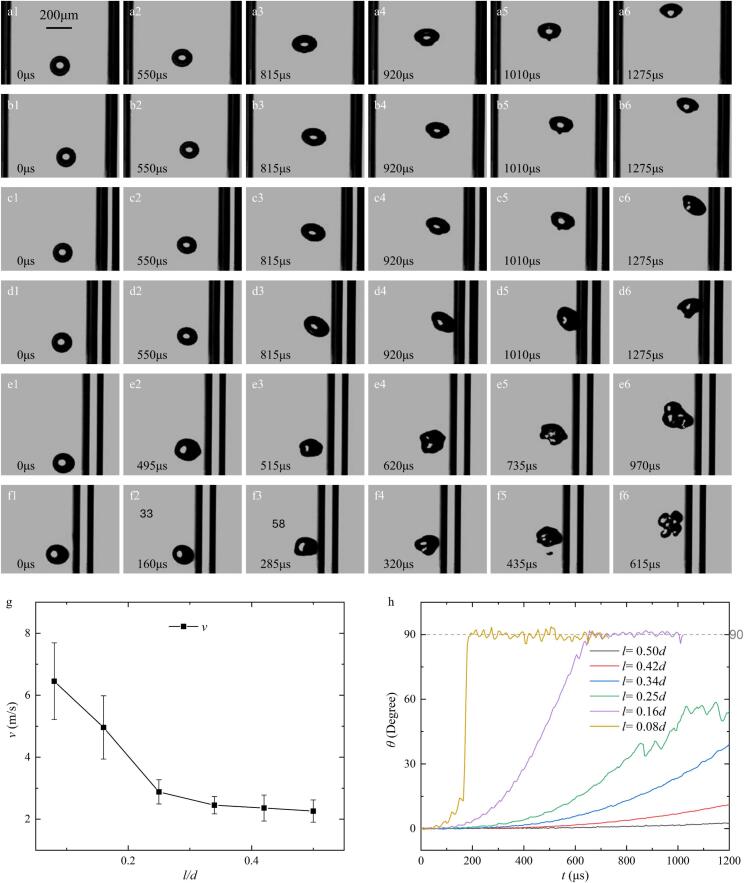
Comparison of the dynamic behaviour of bubbles (*R*_0_ = 75 μm) in different positions inside the tube (*d* = 800 μm, *h* = 200 μm) in an ultrasonic field (*P* = 1.5 kPa, *f* = 16.6 kHz): (a) *l/d* = 0.5. (b) *l/d* = 0.42. (c) *l/d* = 0.34. (d) *l/d* = 0.25. (e) *l/d* = 0.16. (f) *l/d* = 0.08. (g) Velocities of microjets (or the fastest speed of the bubble margin if no microjet occurs) vs. the value of *l/d* (n = 7); (h) The typical angle (*θ*) vs. time *t* for bubbles with various initial value of *l/d*.
